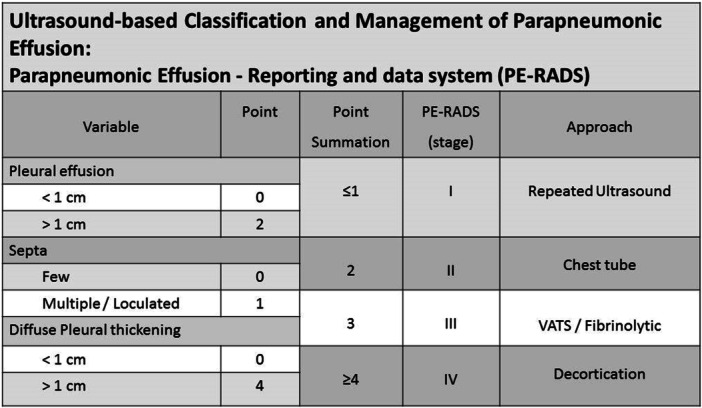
Proposed ultrasound ‐ based classification and management of parapneumonic effusion (Parapneumonic Effusion – Reporting and Data System).
